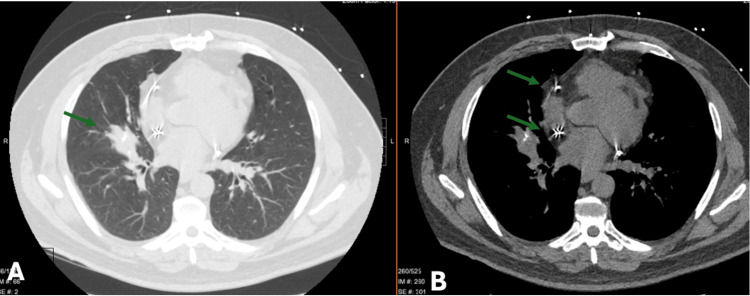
Computed tomography findings of broncholithiasis in Case 3. Axial contrast-enhanced chest CT images displayed in lung window (A) and mediastinal window (B) demonstrate a calcified broncholith obstructing the right middle lobe (RML) bronchus (green arrows). Associated focal airway narrowing is evident, correlating with the patient’s obstructive symptoms.
